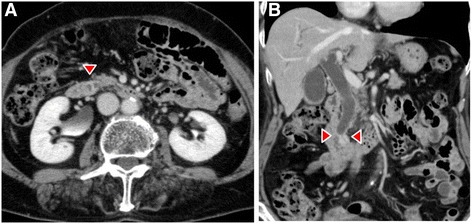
Dynamic CT images, arterial phase, a axial view, and b coronal view show thickening of the wall that is enhanced in the lower part of the CBD (*red arrowheads*), accompanied by a proximal dilatation of the biliary tract. No lymph node metastases are observed
